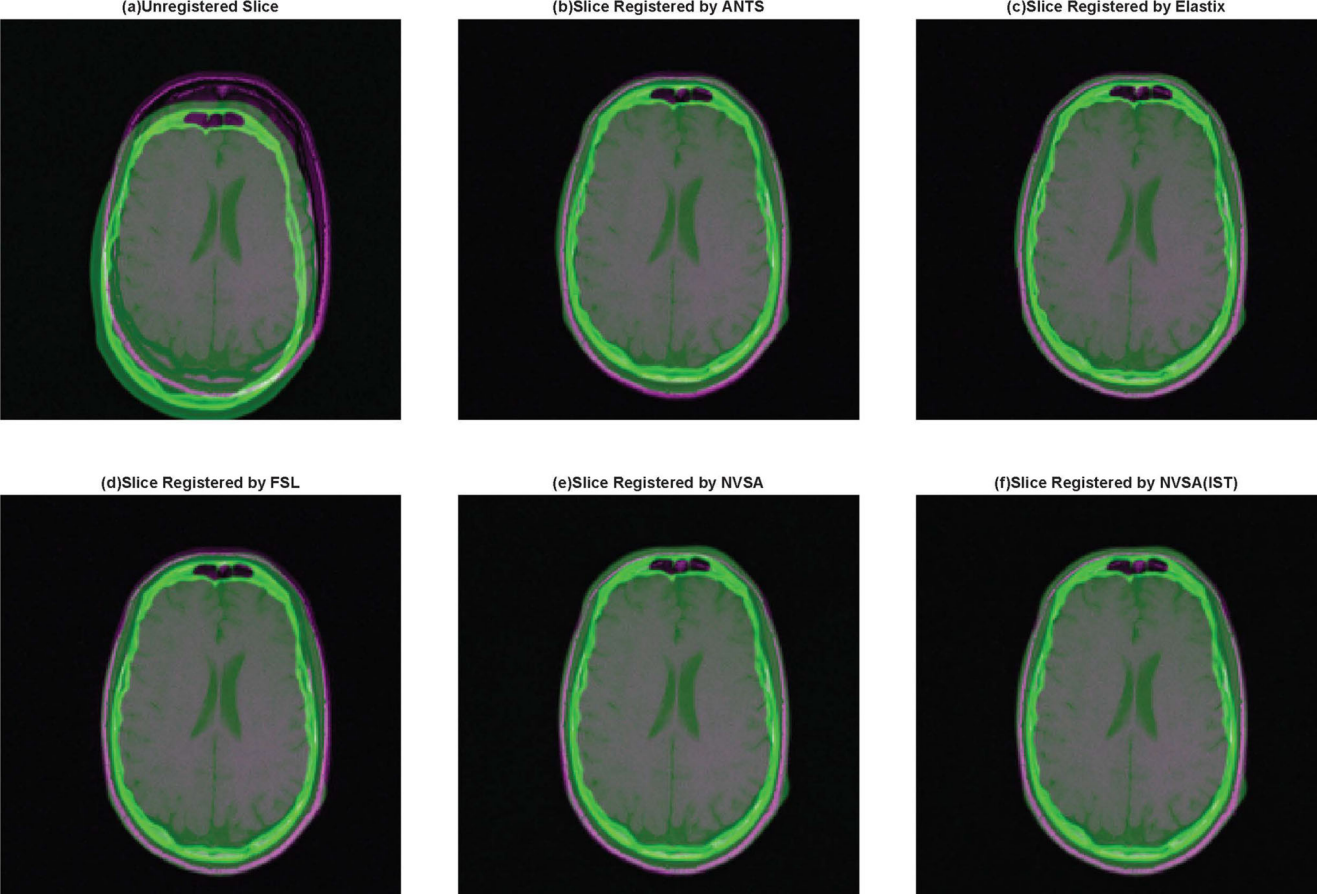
Visualizations of the registration results between multimodal P002 CT-T1 image on three classical methods and NVSA
